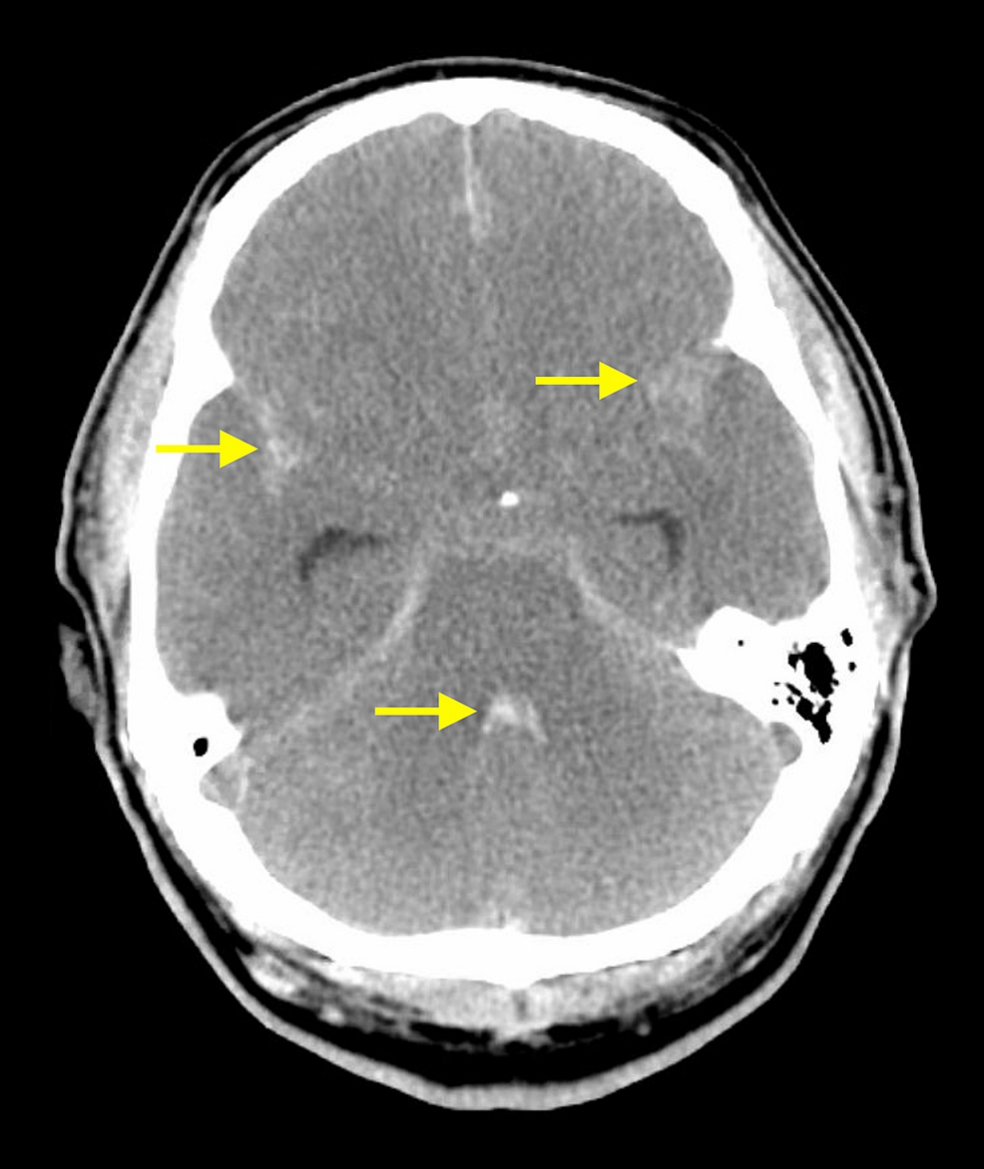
CT imaging reveals hemorrhage within the fourth ventricle (center arrow) and within the anterior subarachnoid spaces bilaterally (lateral arrows) in close proximity to the Sylvian fissure. CT: computed tomography.
